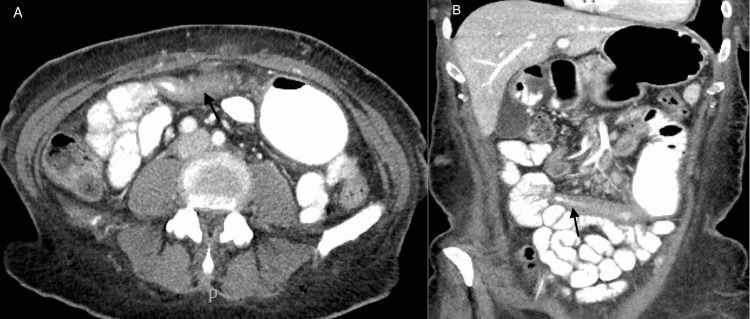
CT of the abdomen with oral contrast: (A) axial and (B) coronal images Concentric wall thickening of the proximal jejunum with stricture formation and proximal dilation.
